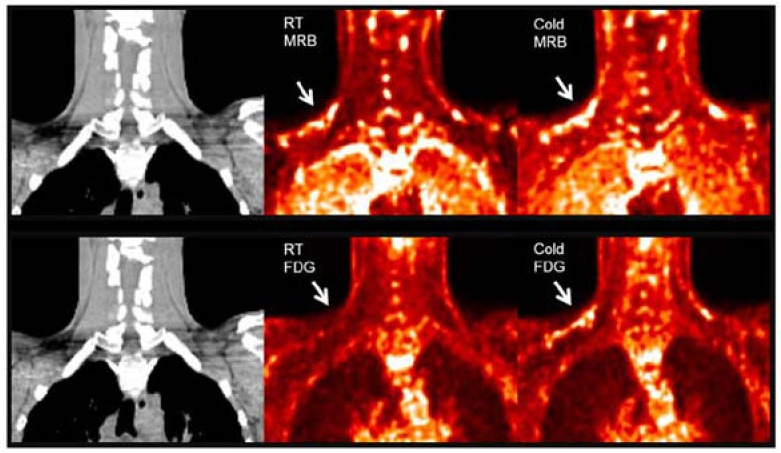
BAT imaging with ^11^C-MRB and ^18^F-FDG under room and cold temperature in male subject. ^18^F-FDG and ^11^C-MRB are scaled from SUV 0 (black) to SUV 2 (white). ^11^C-MRB images are computed from the average of frames acquired between 40 to 60 min post-injection. Original data with permission from reference [54].
